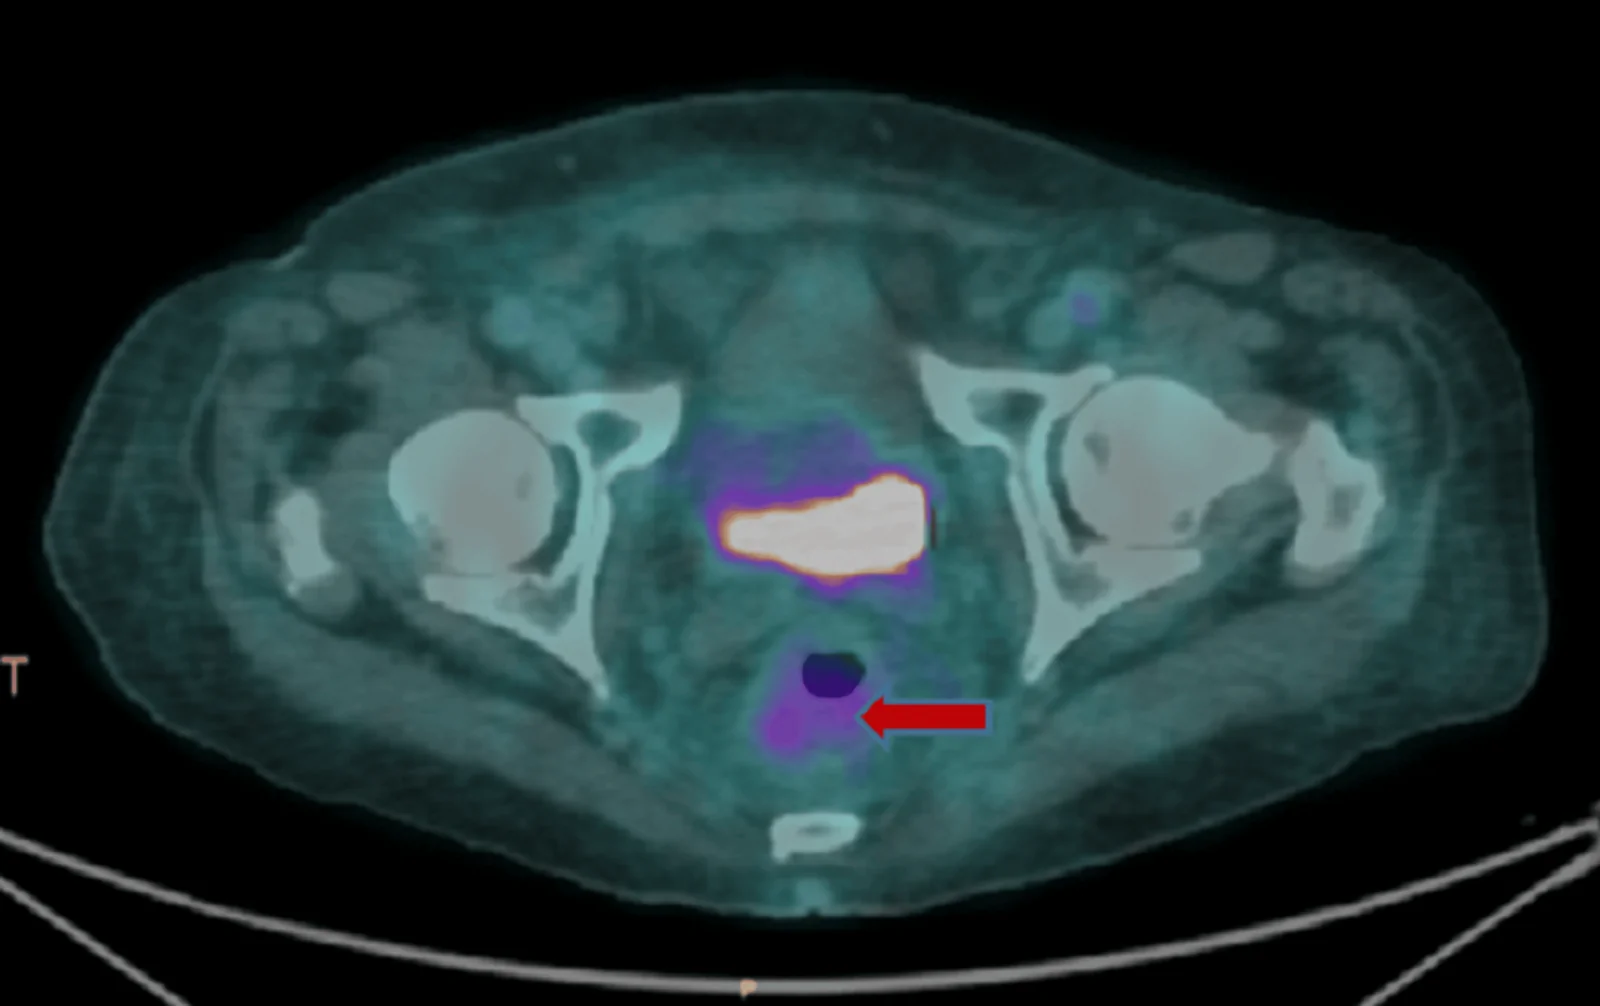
Positron emission tomography computed tomography of the pelvis showing good response of rectal tumor after induction treatment (arrow)
